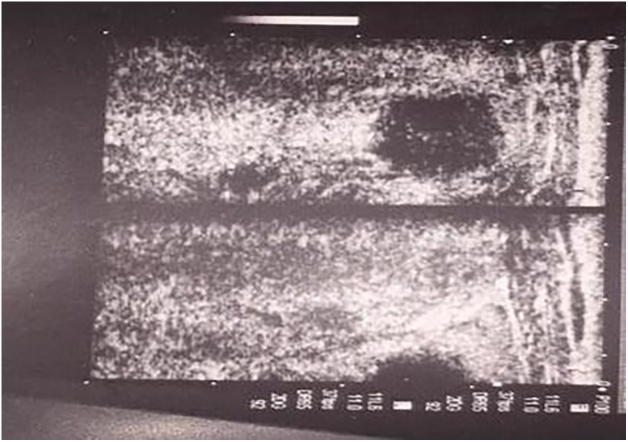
Ultrasound showing cystic lesion at nape of neck.
